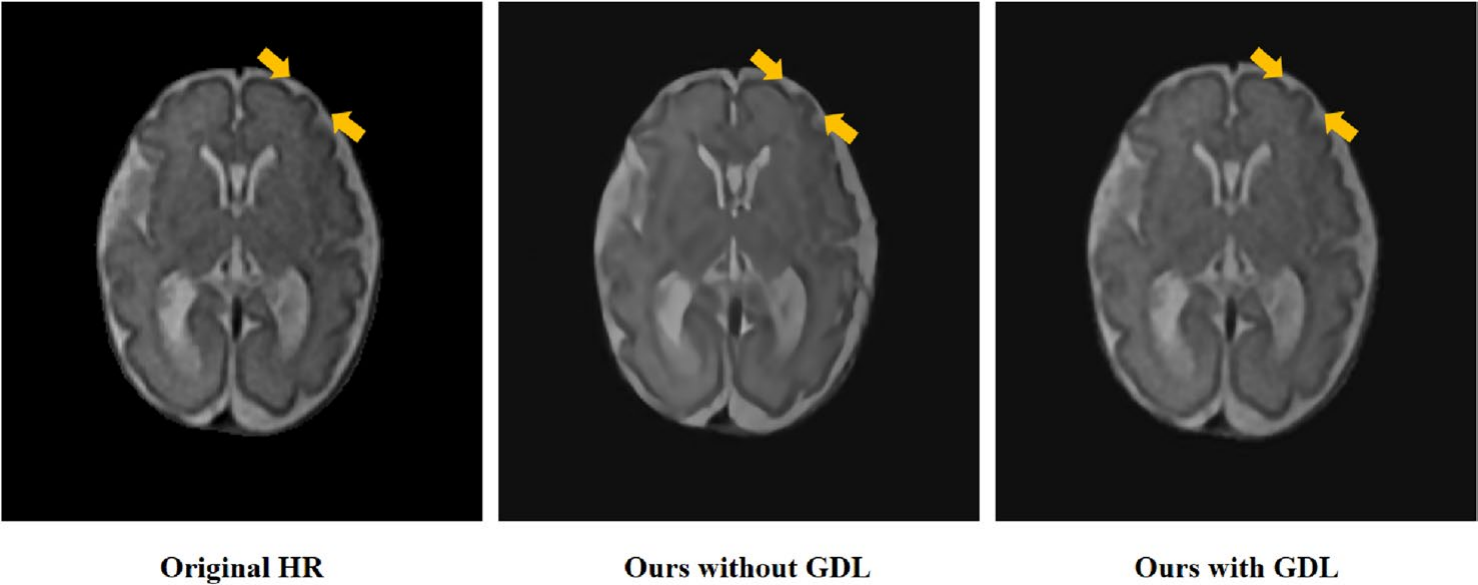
Visual difference between our model with GDL and without GDL on the clinical fetal brain MRI dataset.
